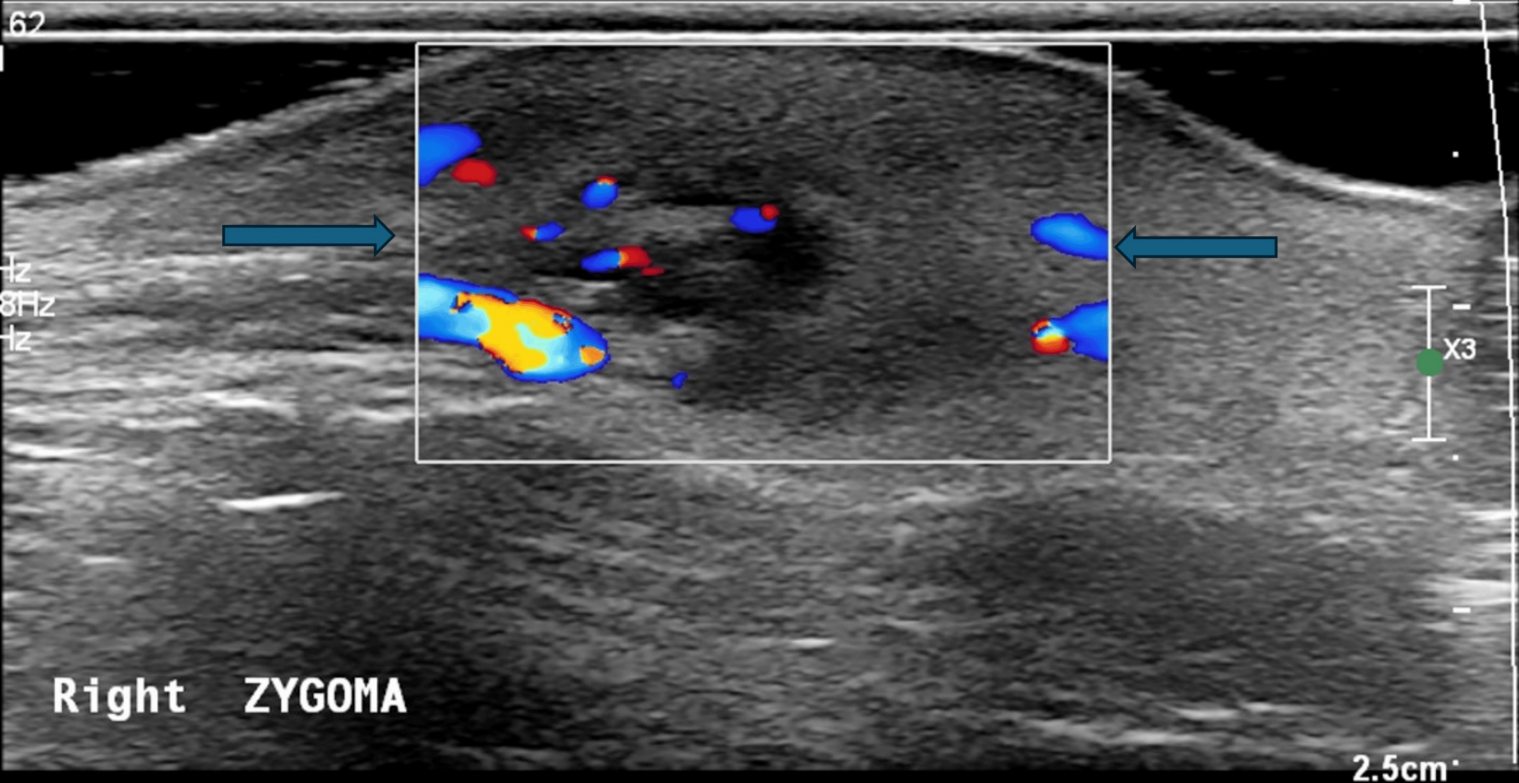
Ultrasonography of the lesion with color Doppler (May 2025) demonstrating increased vascularity within and around the lesion (arrows), which may suggest inflammatory changes.
